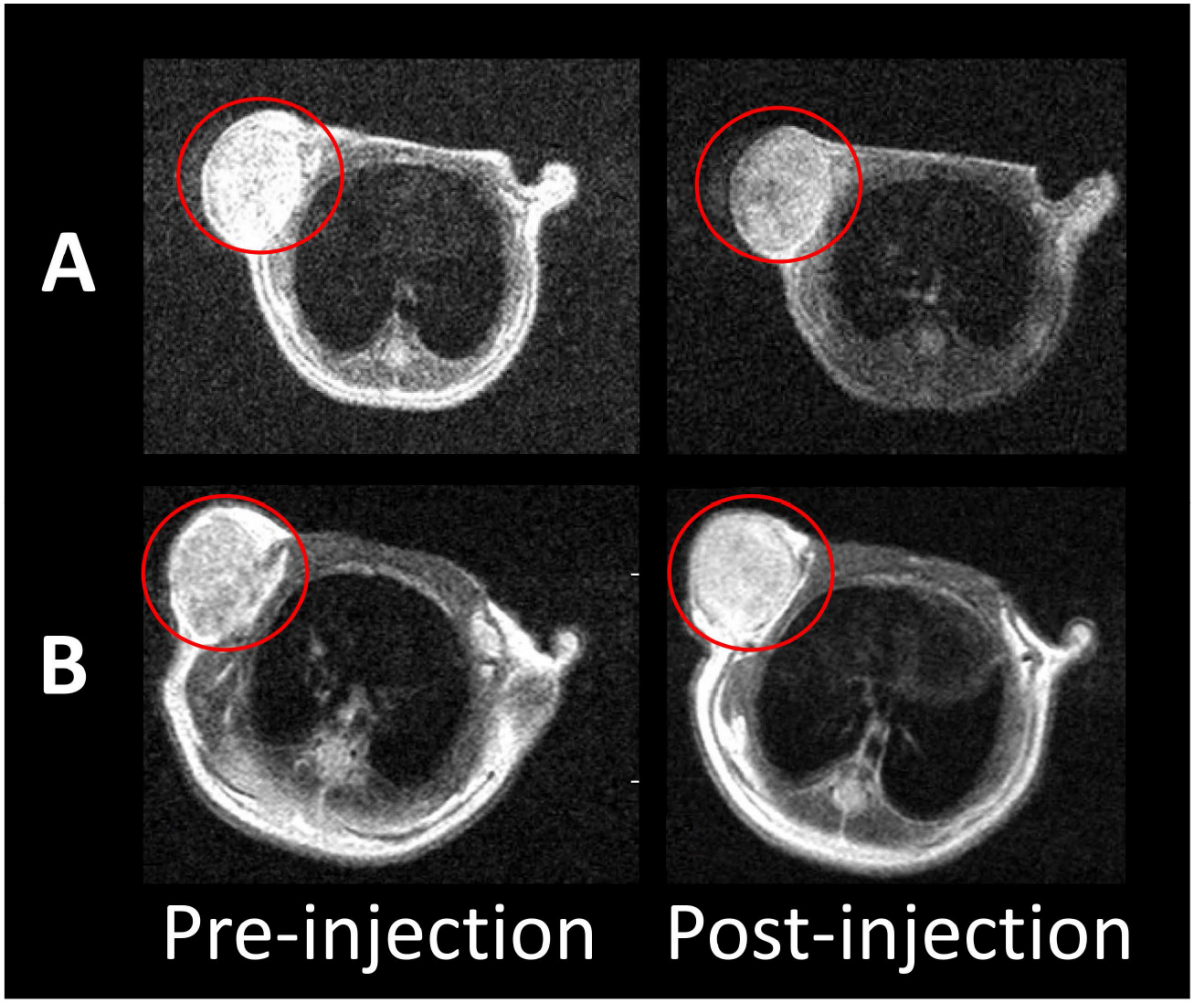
In vivo* T2-weighted MR imaging before and after the injection of SPIO@Liposome-ICG-RGD A. or SPIO@Liposome-ICG B.
